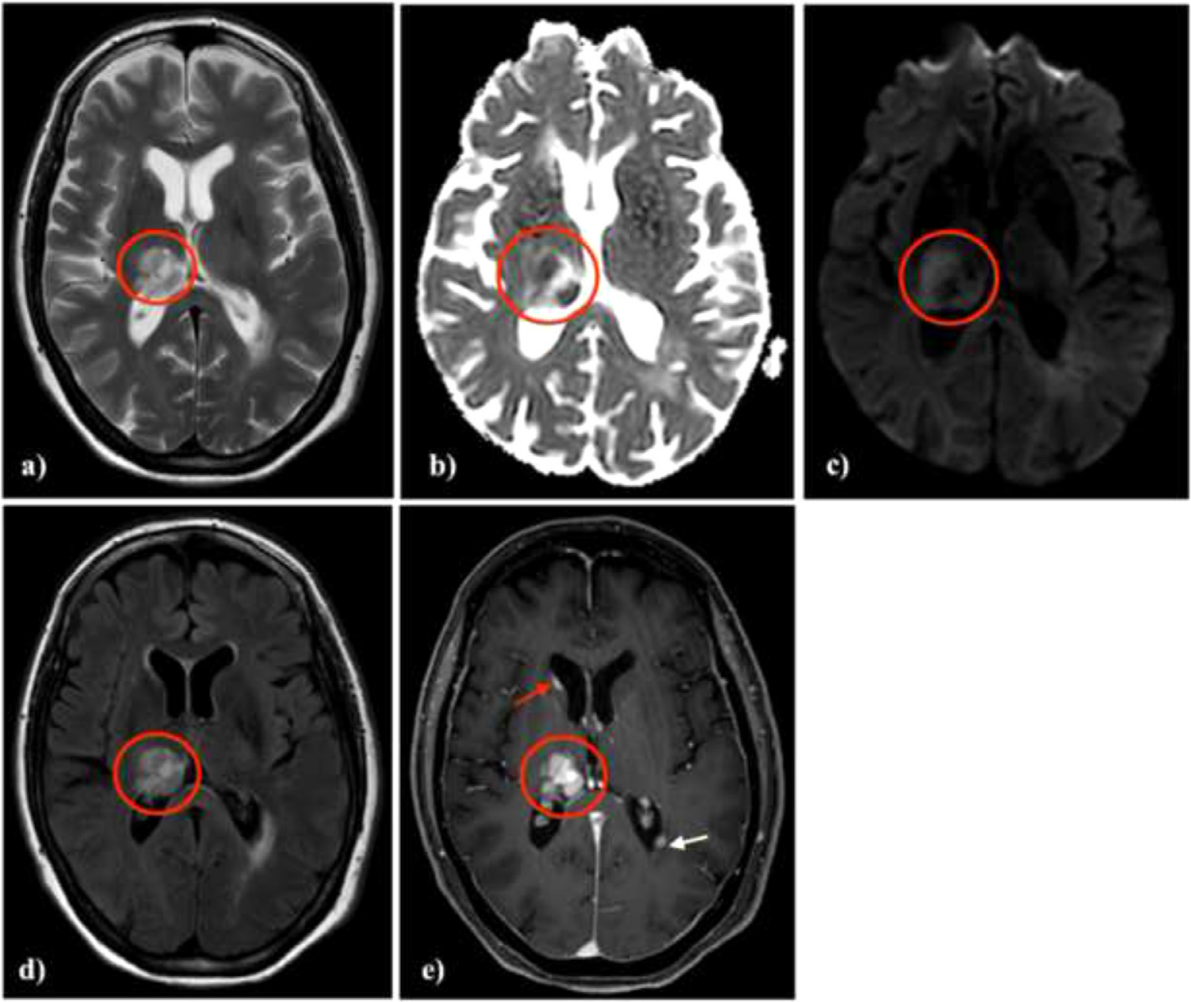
MR imaging: T2-weighted images demonstrate an inhomogeneous slightly hyperintense lesion in the right thalami, with little surrounding vasogenic edema (A, D) and restricted diffusion on DWI (B, C). Axial postcontrast T1-weighted image shows intense contrast enhancement of the thalamic lesion. Other two nodular enhancing lesions are localized in the left peritrigonal and periventricular white matter of the right frontal horn (E).
